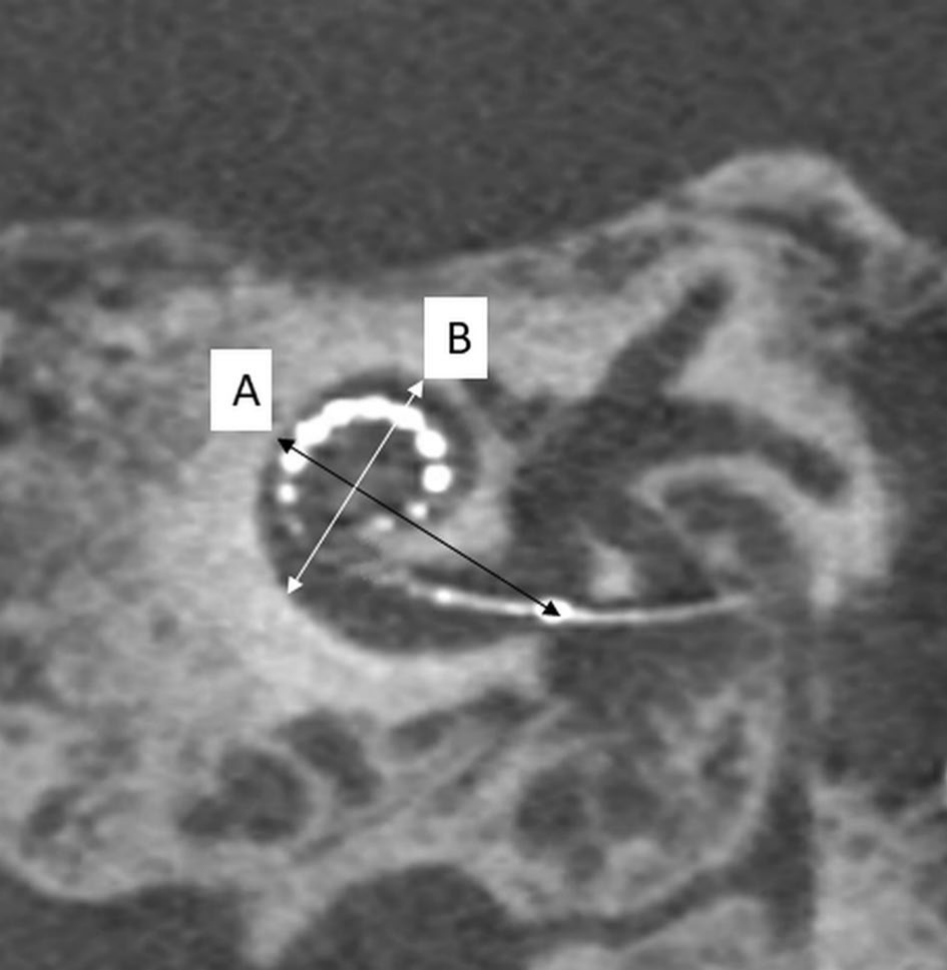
Oblique coronal cone beam CT image demonstrating the distance A and B measurements method. Distance A is indicated by a measurement through the mid-modiolar axis which passes from the round window (at the location of the reference electrode) to the diametrically opposite outer wall of the basal turn of cochlear. Distance B is measured perpendicular to distance A through the mid-modiolar axis, extending between the outer walls of the inferior and superior segments of the basal turn.
